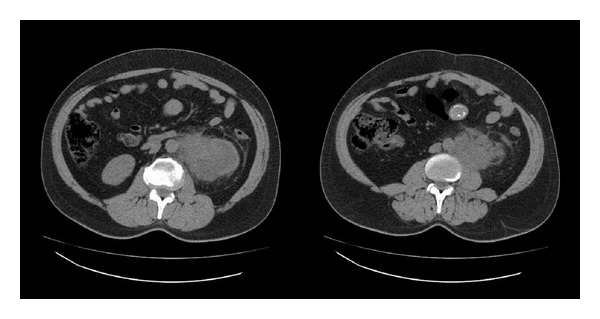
8.5 × 7.5 cm lymph node surrounding the left proximal ureter and the abdominal aorta in the left para-aortic area on noncontrast computed tomography.
